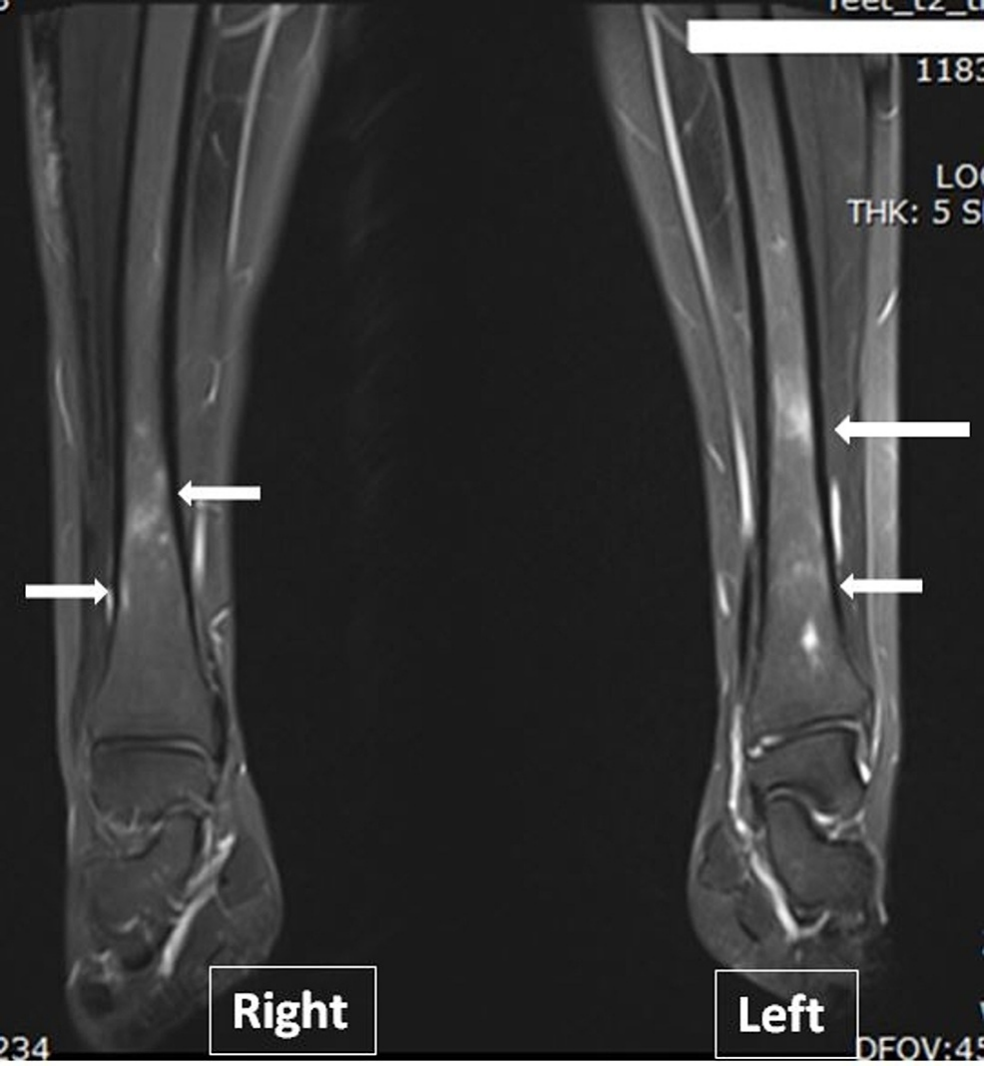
Selected STIR sequence of MRI both legs. It shows high signal intensity multiple lesions involving both tibias. STIR, short tau inversion recovery
